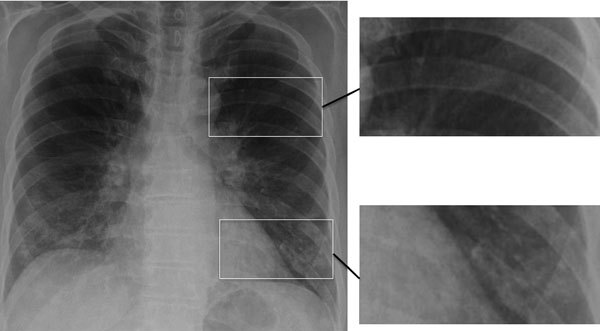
Chest radiograph showing early, subtle *Pneumocystis* pneumonia–associated abnormalities in both lower lungs of a patient newly diagnosed with AIDS; this diagnosis was unsuspected in the patient, a 63-year-old married man. Magnified images on right show normal lung (top image) and infiltrates adjacent to and behind the heart and overlain by rib (bottom image). Similar differences between the upper and lower lobes are seen in the radiograph on the left. Image used with permission of David Denning (©2016, all rights reserved).
